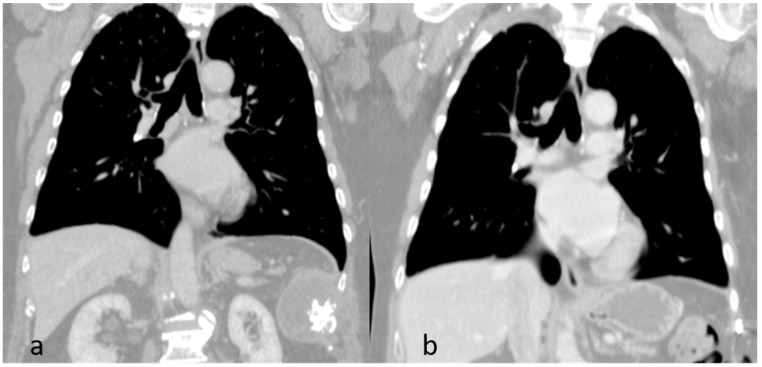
Coronal view computed tomography scans: Pre-operative finding (a). Post-operative finding (b).
